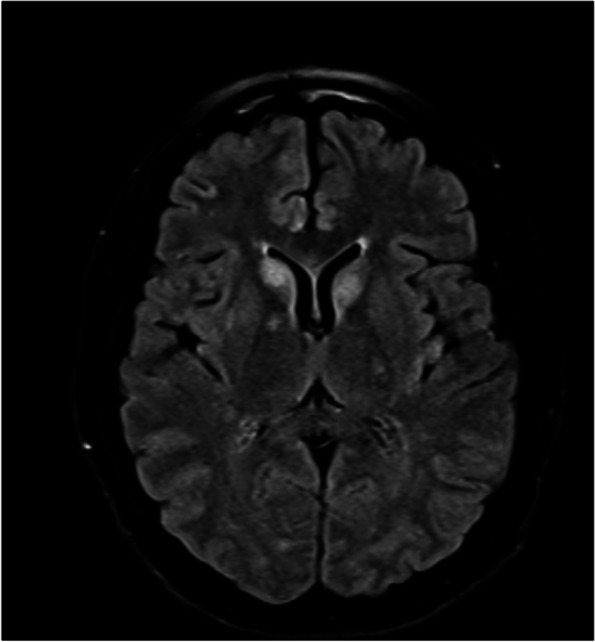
Brain magnetic resonance imaging: T2/FLAIR sequence finding well-defined focal hyperintense lesions at the bilateral caudate nucleus, and demyelinating lesions at the bilateral subcortical areas, already observed in a previous imaging study
